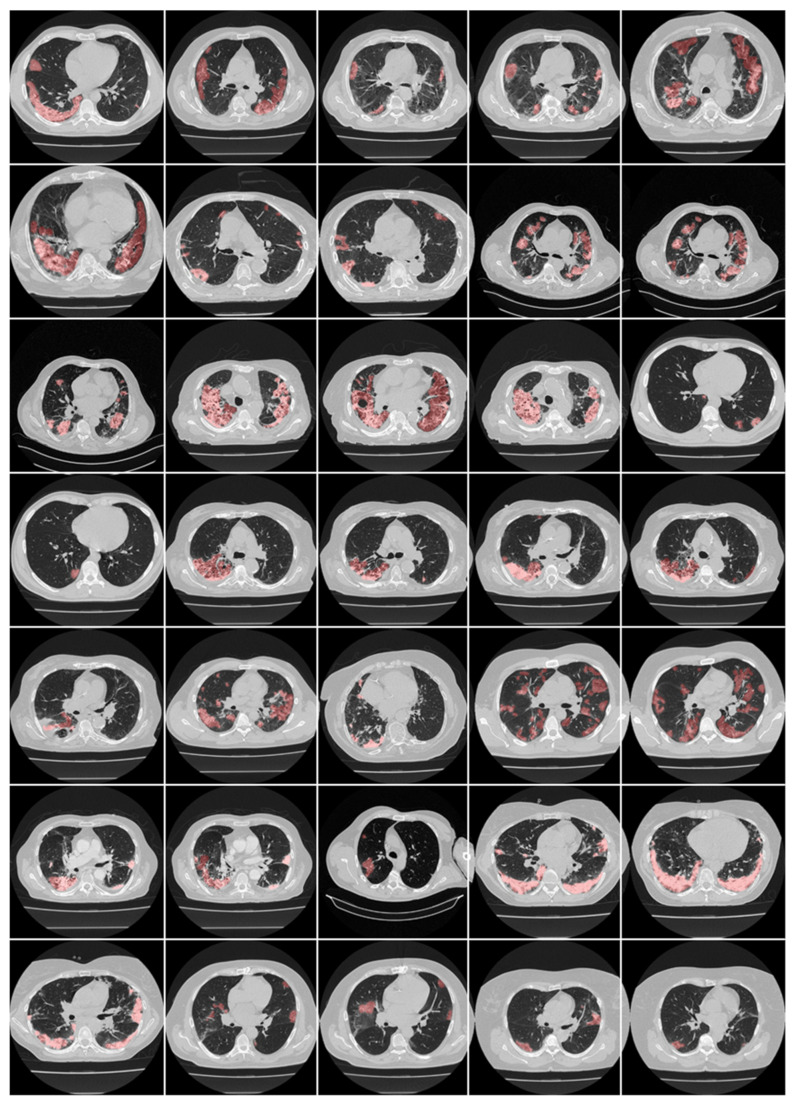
Manual lesion delineation overlays (red) from tracer 2 on raw CT lung images (Courtesy of AtheroPoint™, Roseville, CA, USA permission granted).
